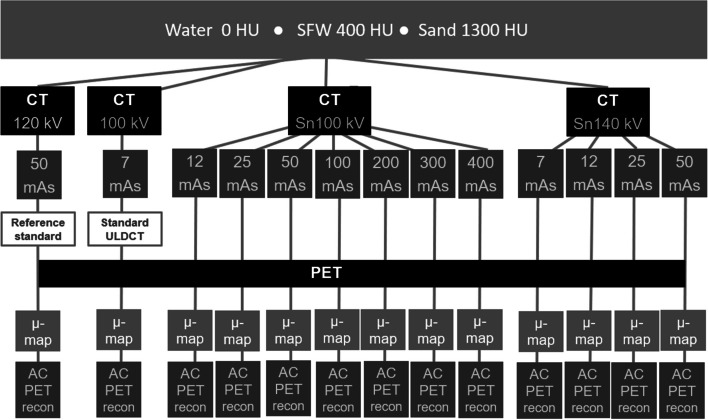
Overview of the CT and PET datasets generated in this study. AC = attenuation correction, HU = Hounsfield unit, mAs = milliampere seconds, SFW = sand + flour + water, ULDCT = ultra-low-dose CT
